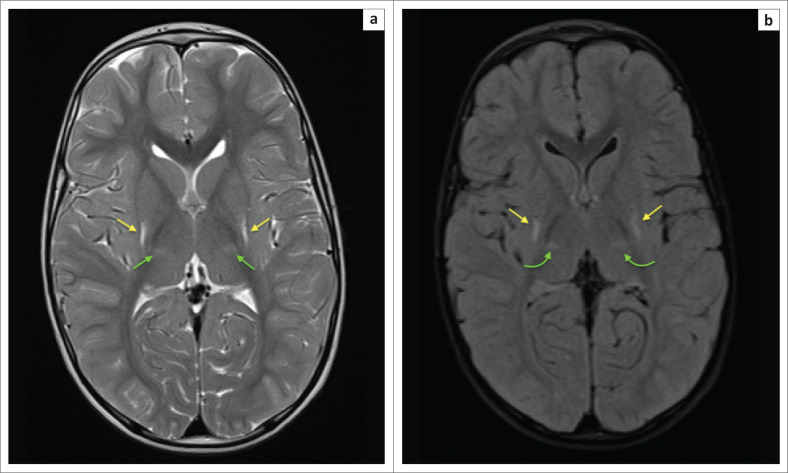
Four-year-old male child with cerebral palsy. Neonatal history of hypoxic ischemic brain injury-related encephalopathy, seizures, requiring ventilation and prolonged 4 week stay in ICU. (a) An axial T2-weighted sequence and (b) an axial FLAIR sequence image showing subtle flame shaped dorsal putaminal (yellow arrows) hyperintensity and smudge-like ventral thalamic (green arrows) hyperintensity bilaterally.
